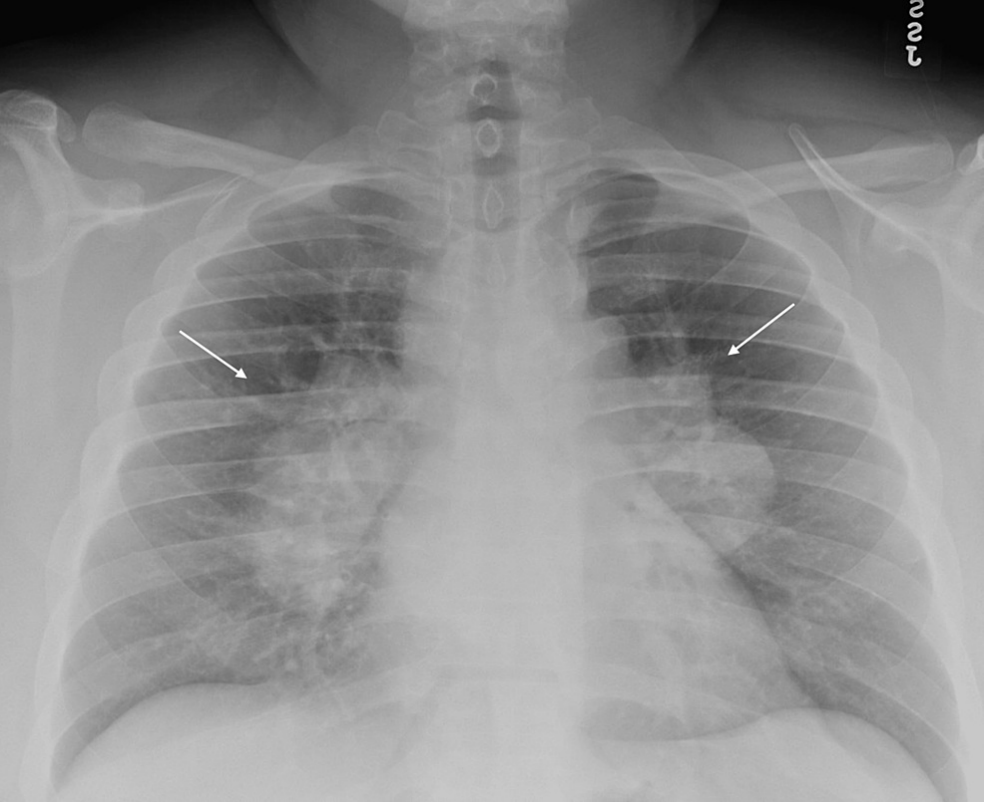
Chest X-ray The image is demonstrating severe mediastinal and bilateral lymphadenopathy.
